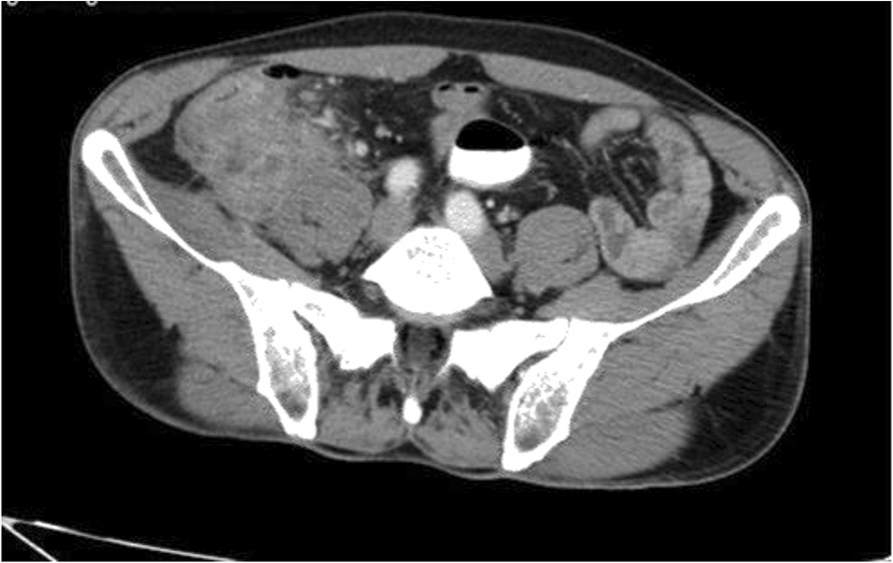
Cecal Diverticulitis: Axial pre-contrast CT image shows mesenteric inflammation adjacent to the distal ileum and cecum, minimal free peritoneal fluid and free air wall thickening and multiple small diverticula in the distal ileum.
